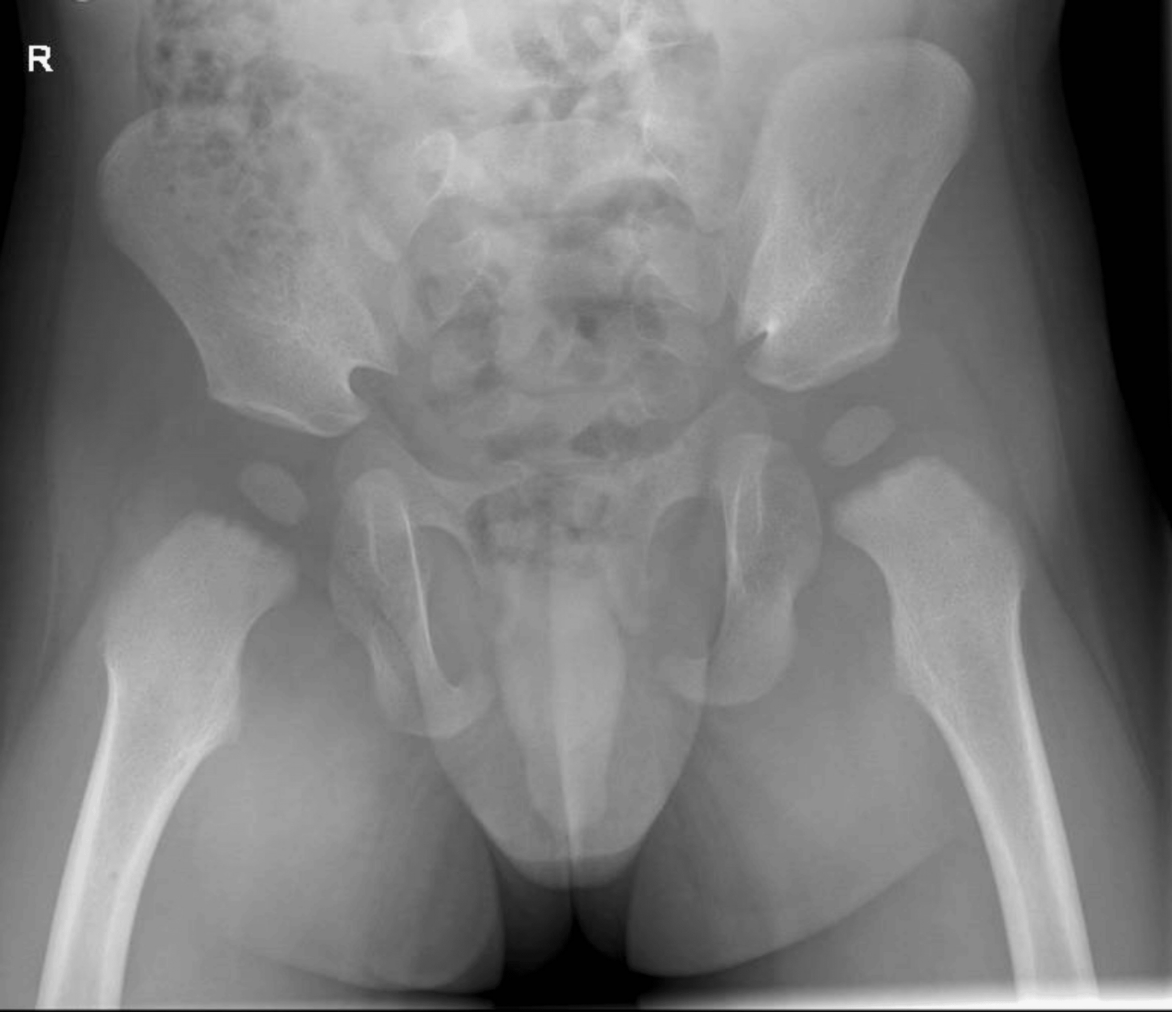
An X-ray of the pelvis shows the femoral capital epiphyses are symmetrical in height and density. Both femoral heads are appropriately covered by their respective normal morphology acetabula. Allowing for minor rotation, there is normal alignment of both hip joints.
